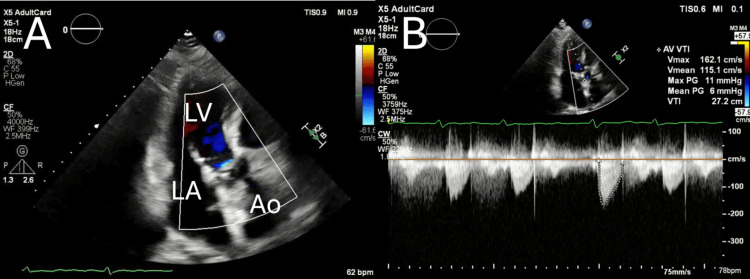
Postoperative transthoracic echocardiography (TTE). (A) Color Doppler imaging demonstrates only trivial transvalvular leakage following valve implantation. (B) Continuous-wave Doppler across the aortic valve shows a peak velocity in the 1 m/s range, with a maximum pressure gradient of 11 mmHg and a mean pressure gradient of 6 mmHg.
